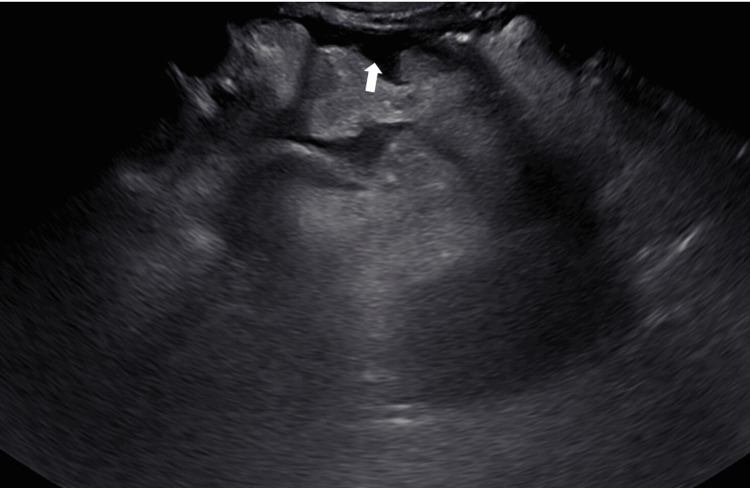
Repeat abdominal ultrasound showing resolution of loculated ascites post-tPA therapy, indicated by an arrow. tPA: Tissue Plasminogen Activator.
